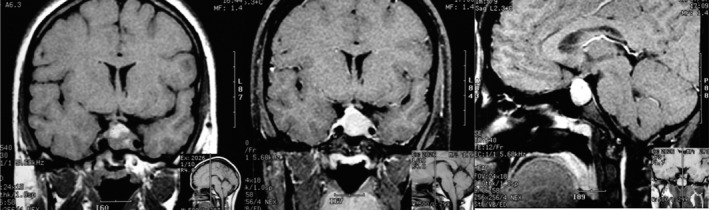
Coronal and sagittal T1 weighted pituitary MRI image at diagnosis shows a sellar mass with optic chiasmal compression and left cavernous sinus invasion.
